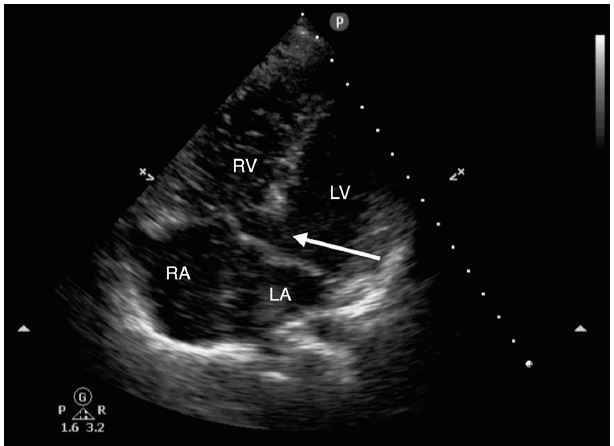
Apical four chamber view, slightly off axis, with a large ventricular septal defect (arrow). *RV*, right ventricle; *LV*, left ventricle; *RA*, right atrium; *LA*, left atrium
